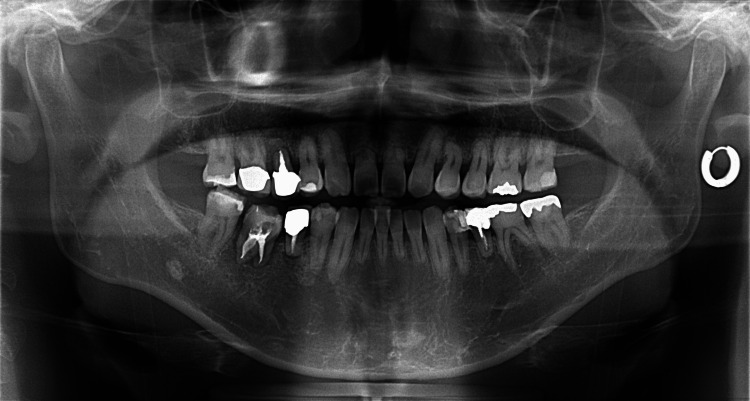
Panoramic radiograph after root canal treatment
